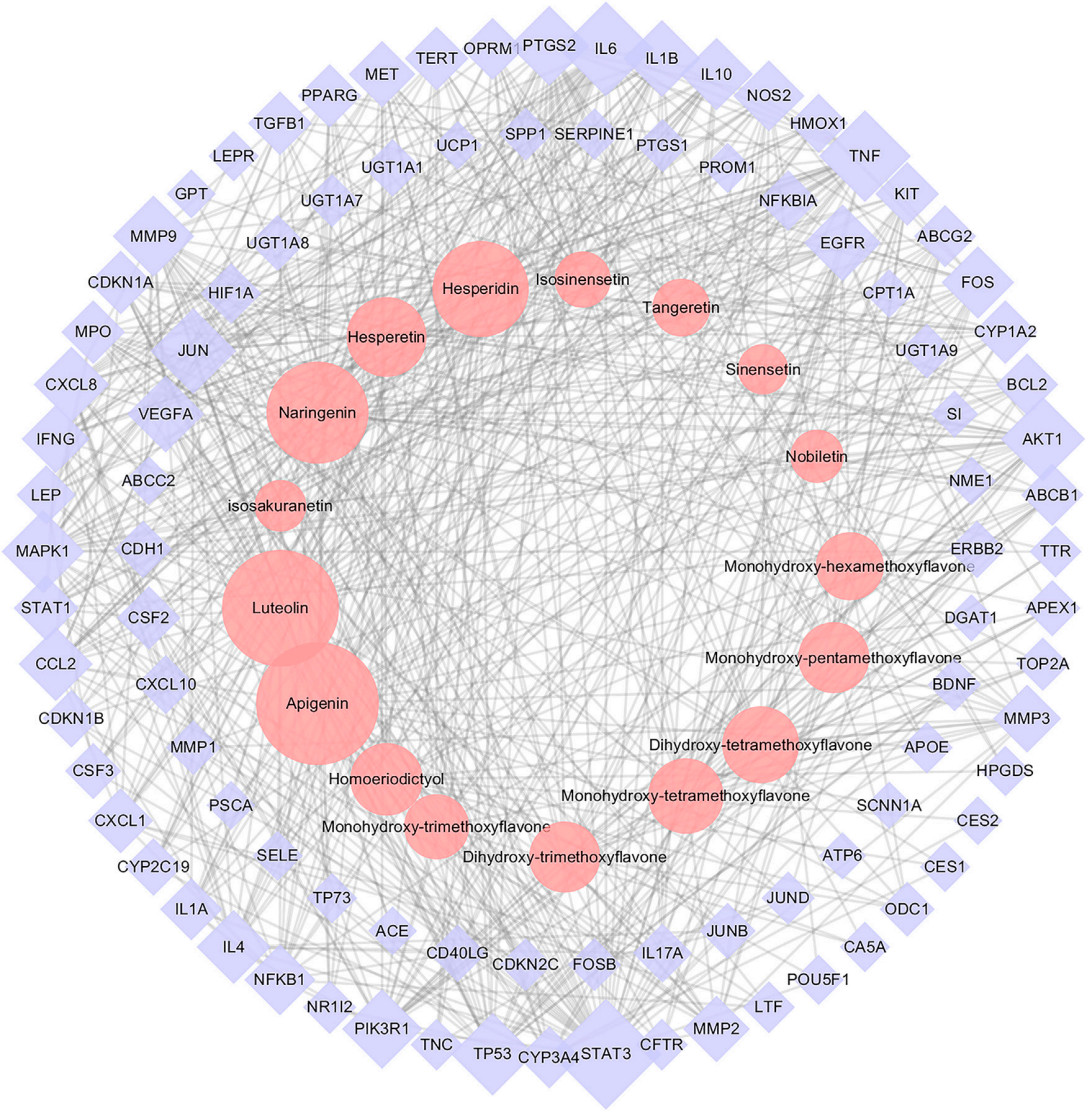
The Citri Reticulatae Pericarpium (CRP)-related target-spleen deficiency target network (CT-ST network). Pink circles, purple diamonds represented CRP compounds and the common targets for both compounds and spleen deficiency, respectively. The size of circles and diamonds indicated nodes degree value.
